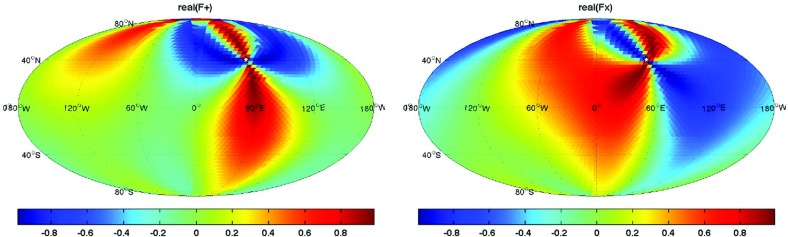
Mollweide projections of the response functions $$R_\mathrm{doppler}^+(\hat{n})$$, $$R_\mathrm{doppler}^\times (\hat{n})$$, for one-way tracking Doppler frequency measurements corresponding to a pulsar located in the direction of the white star $$(\theta ,\phi )=(50^\circ , 60^\circ )$$. The imaginary parts of both response functions are identically zero, so are not shown above
